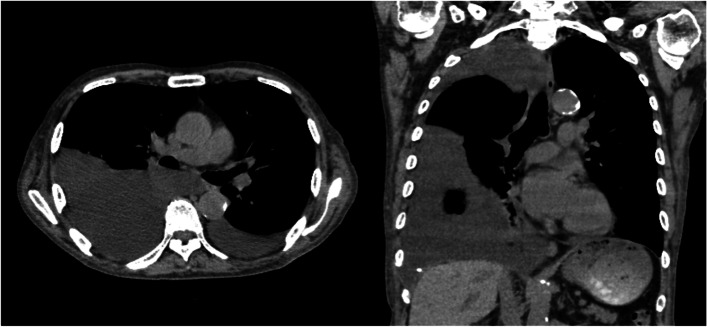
Computed tomography scan showing a uniform right massive pleural effusion
